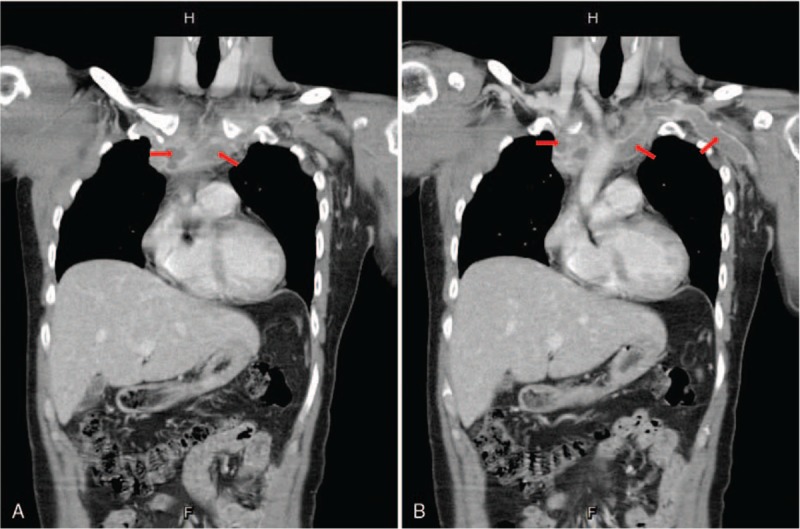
(A, B) Computed tomographic angiography image showing venous thrombosis involving bilateral subclavian veins.
